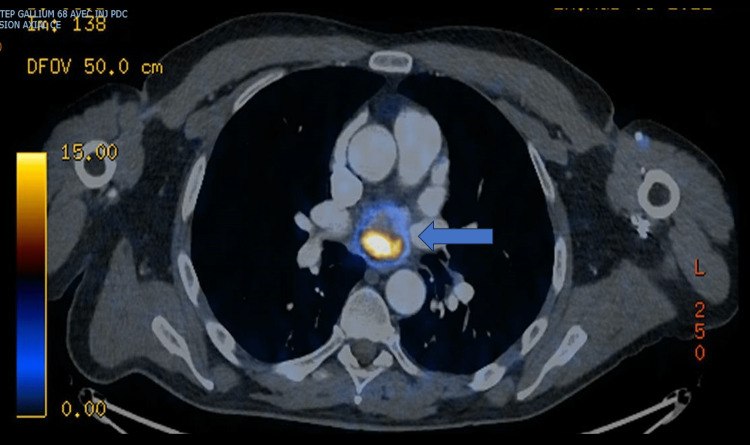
Left atrial mass during positron emission tomography/computed tomography
